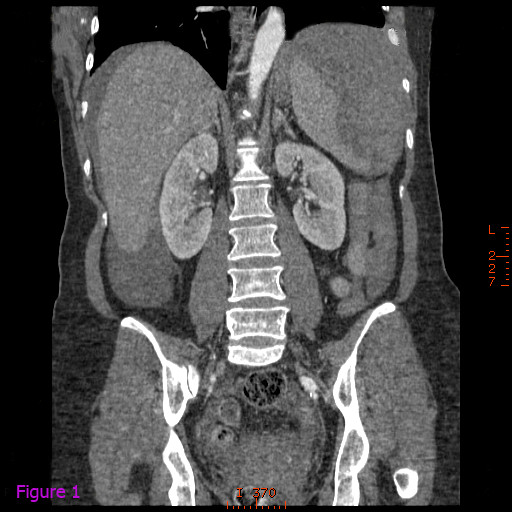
Contrast-enhanced computed tomographic scan of the abdomen revealing splenic subcapsular hematoma with loss of normal structure and normal density of the lower pole, consistent with acute spontaneous splenic rupture.
